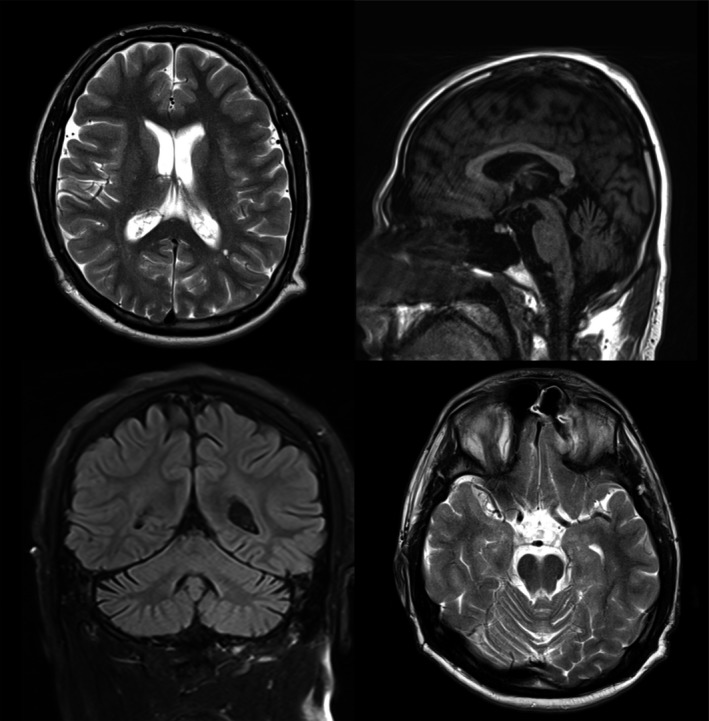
MRI imaging of Patient 1 demonstrates mild cerebellar atrophy with preservation of the cerebral and brainstem volumes and normal white matter signal.
